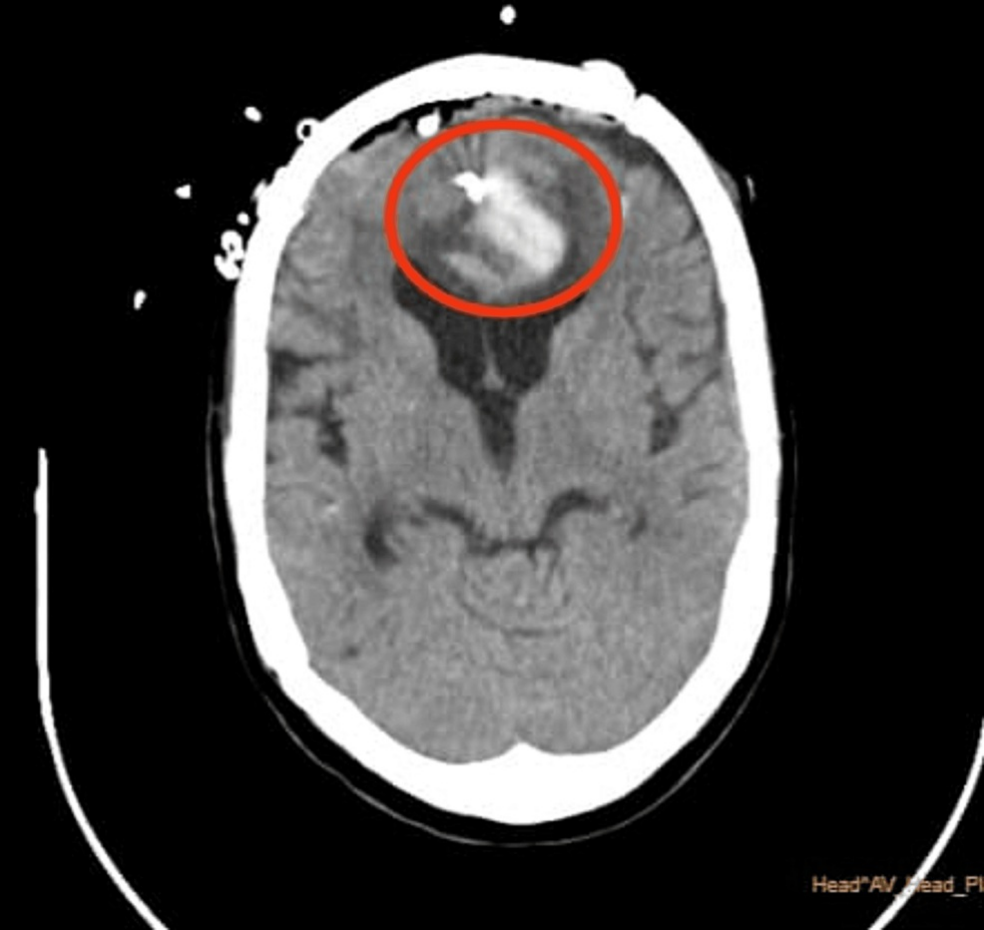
Preoperative computed tomography scan. The red circle shows the DACA aneurysm. DACA: distal anterior cerebral artery
